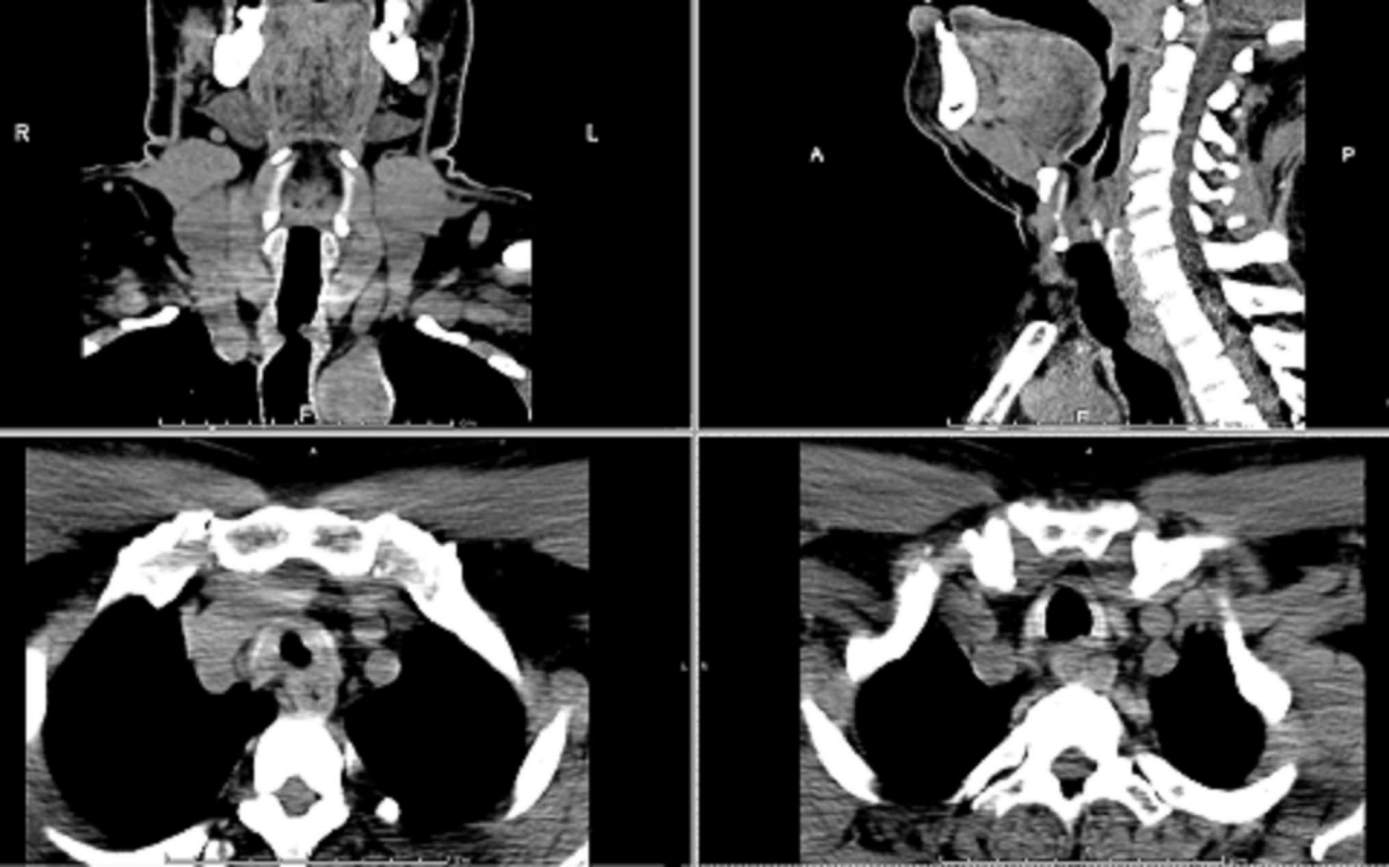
CT scan with multiplanar reconstructions of a patient affected by tracheal benign stenosis
